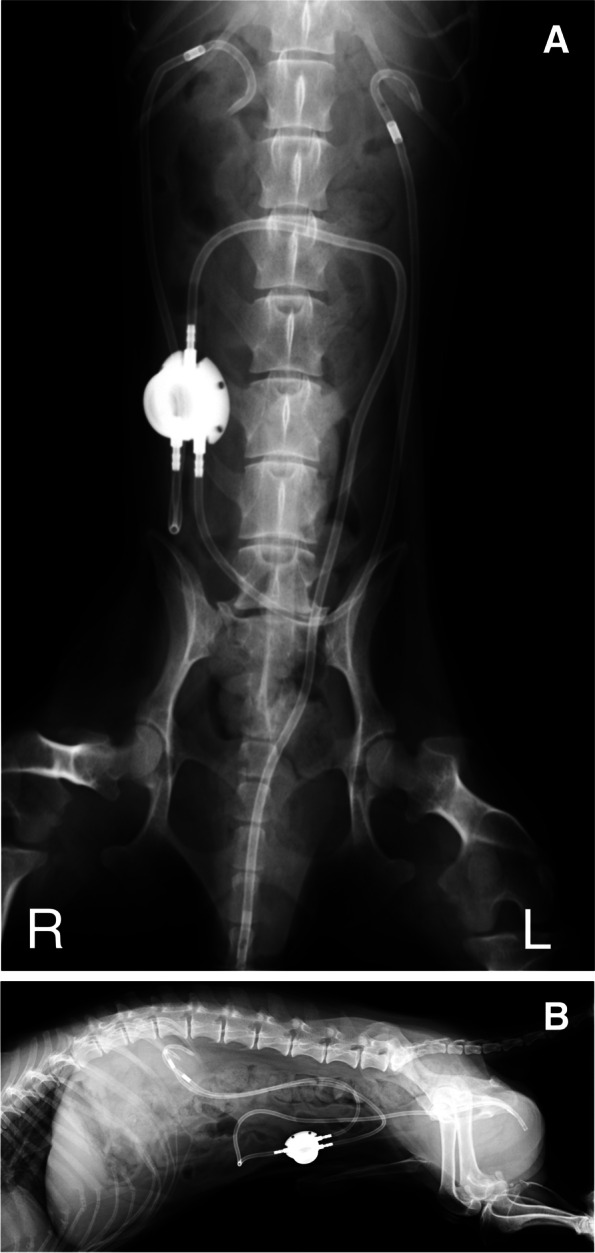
Postoperative radiographs of subcutaneous ureteral bypass system between kidney and urethra with one pantport. (A) Ventrodorsal image. (B) Lateral image
